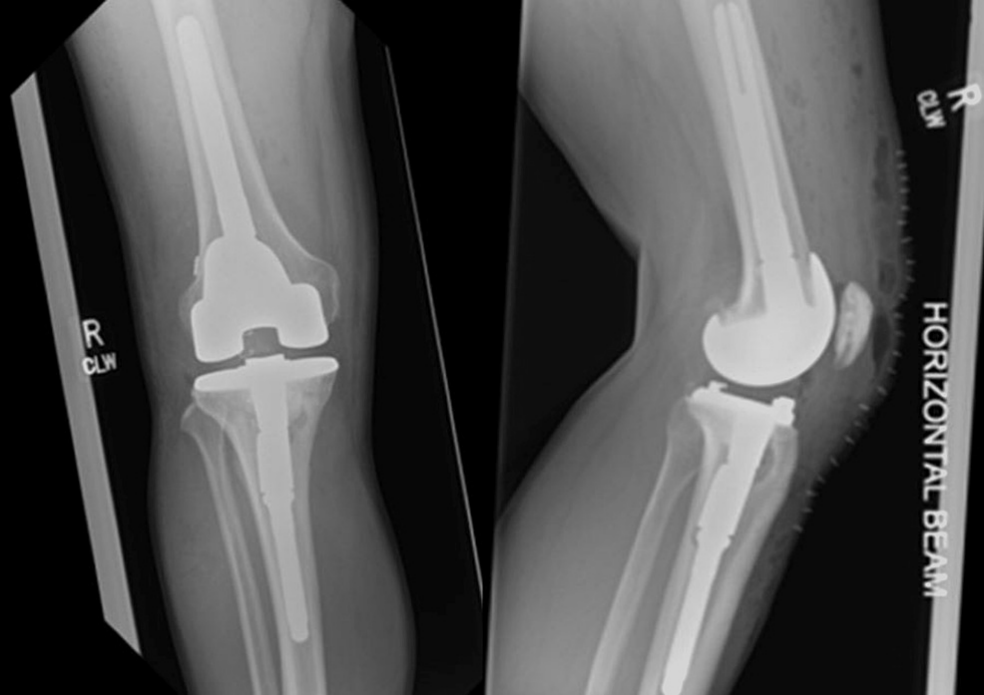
Post-operative radiographs following revision total knee arthroplasty. Antero-posterior (left) and lateral (right) views done in November 2019.
